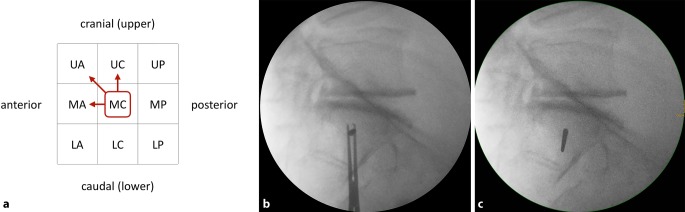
Assessment of the starting point in the lateral view. Three views are mandatory for fluoroscopically controlled SI screw fixation: lateral view, inlet view and outlet view. The techniques for obtaining correct lateral, inlet and outlet views have been previously described in this journal [6]. In addition, the ideal angles for intraoperative fluoroscopic inlet and outlet views can be estimated from preoperative midsagittal computed tomography (CT) reconstructions [4]. It has to be kept in mind that ideal fluoroscopic inlet and outlet view angles are not orthogonal to each other and that the arc of angulation between both views rather averages about 67° [8]. a 55-year-old man with a minimally displaced transforaminal sacral fracture after a fall from height. The preoperative assessment shows no signs of sacral dysmorphism with a safe transverse MC corridor (i. e. both the starting point and the endpoint are located in the MC zone in the lateral view). There is also a safe inlet-oblique corridor (MC—MA), a safe outlet-oblique corridor (MC—UC) and an inlet-outlet-oblique corridor (MC—UA). It is planned to perform an SI screw fixation via a transverse MC corridor. b In the lateral view a central MC starting point is chosen for a planned strict transverse screw trajectory (MC). c The guide wire is advanced to the SI joint for a secure wire purchase in the bone. This additionally allows for assessing the wire trajectory in a lateral view. In the present case fluoroscopic control shows a slight cranial deviation from the planned strict transverse trajectory. Since the outlet-oblique corridor (MC—UC) was preoperatively assessed as safe as well, this trajectory is accepted for further advancement of the wire. *U* upper, *M* middle, *L* lower, *A* anterior, *C* central, *P* posterior
